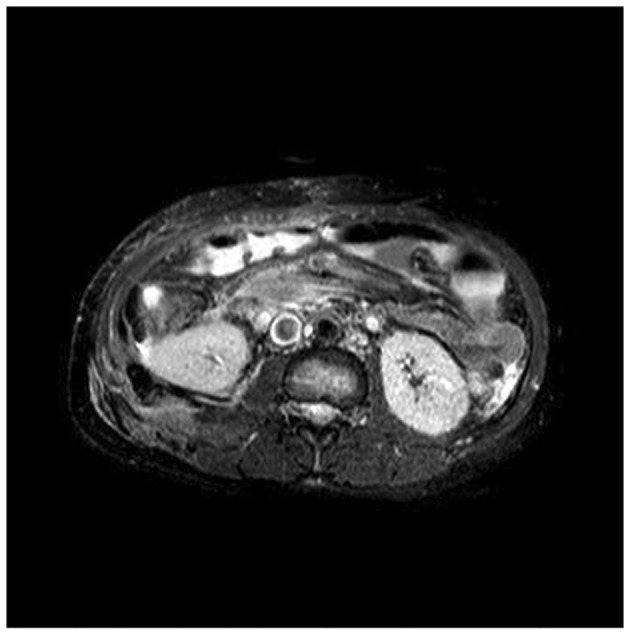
Magnetic resonance imaging showing postsurgical examination of the abdomen.
